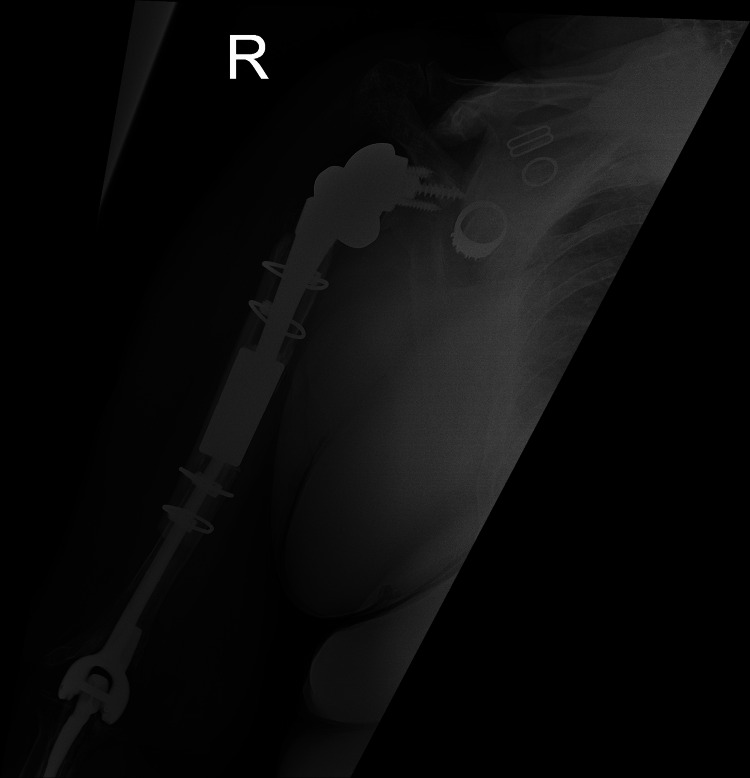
External rotation radiograph of the right humerus at 12-month follow-up.
